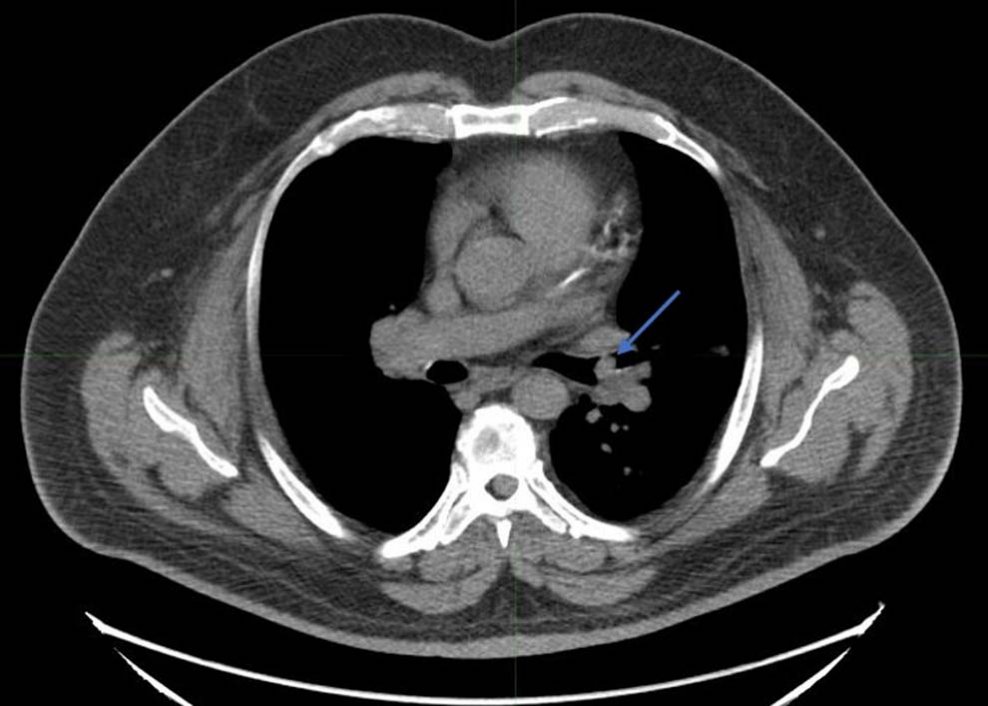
Noncontrast axial chest computed tomography image demonstrates a 1.3-cm soft-tissue endobronchial lesion within the left upper lobe bronchus, concerning for a primary bronchial tumor or metastasis (arrow).
